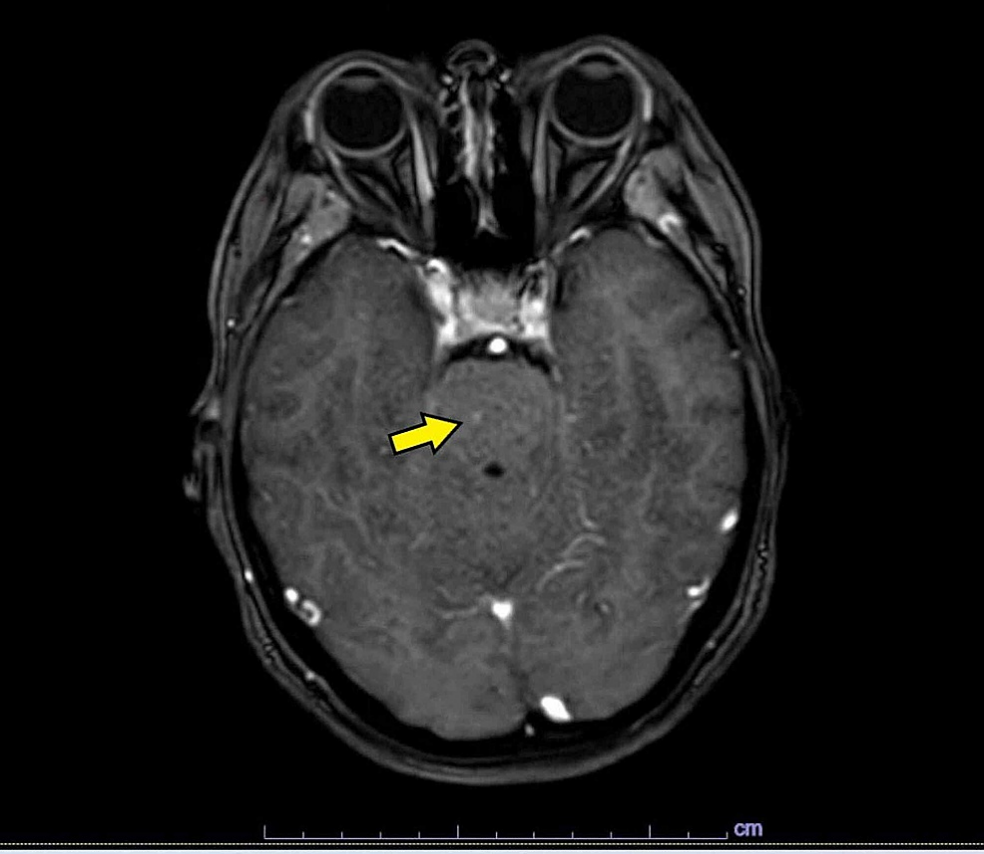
MRI brain. Gadolinium-enhanced T1-weighted axial image showing punctate focus of enhancement at right pons (yellow arrow).
